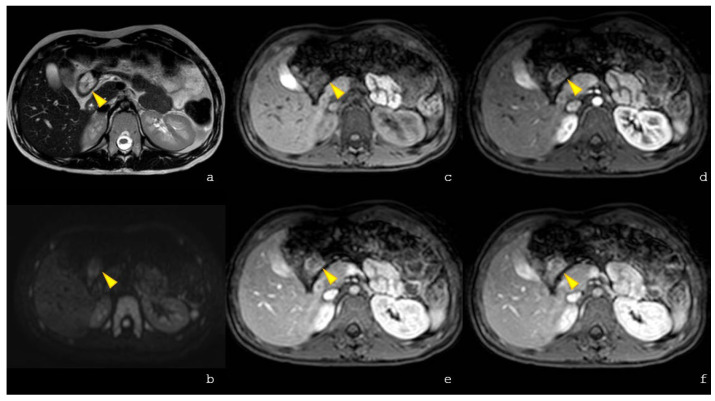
MRI examination in proband 1: Axial T2-weighted imaging (a) confirms the presence of a hyperintense nodular formation with well-defined margins, localized within the colonic lumen at the hepatic flexure indicated by the yellow arrowhead. The lesion shows no restricted diffusion (b), is characterized by no hyperintense signal intensity on pre-contrast axial eTRHIVE images (c) and shows early contrast enhancement during the arterial phase (d), persisting in subsequent phases (e,f). Coronal SPAIR T2-weighted images show the presence of a parapelvic cyst (diameter 11 mm) at the middle of the right kidney (e) and cortical cyst (diameter 5 mm) at the lower pole of the homolateral kidney (f).
